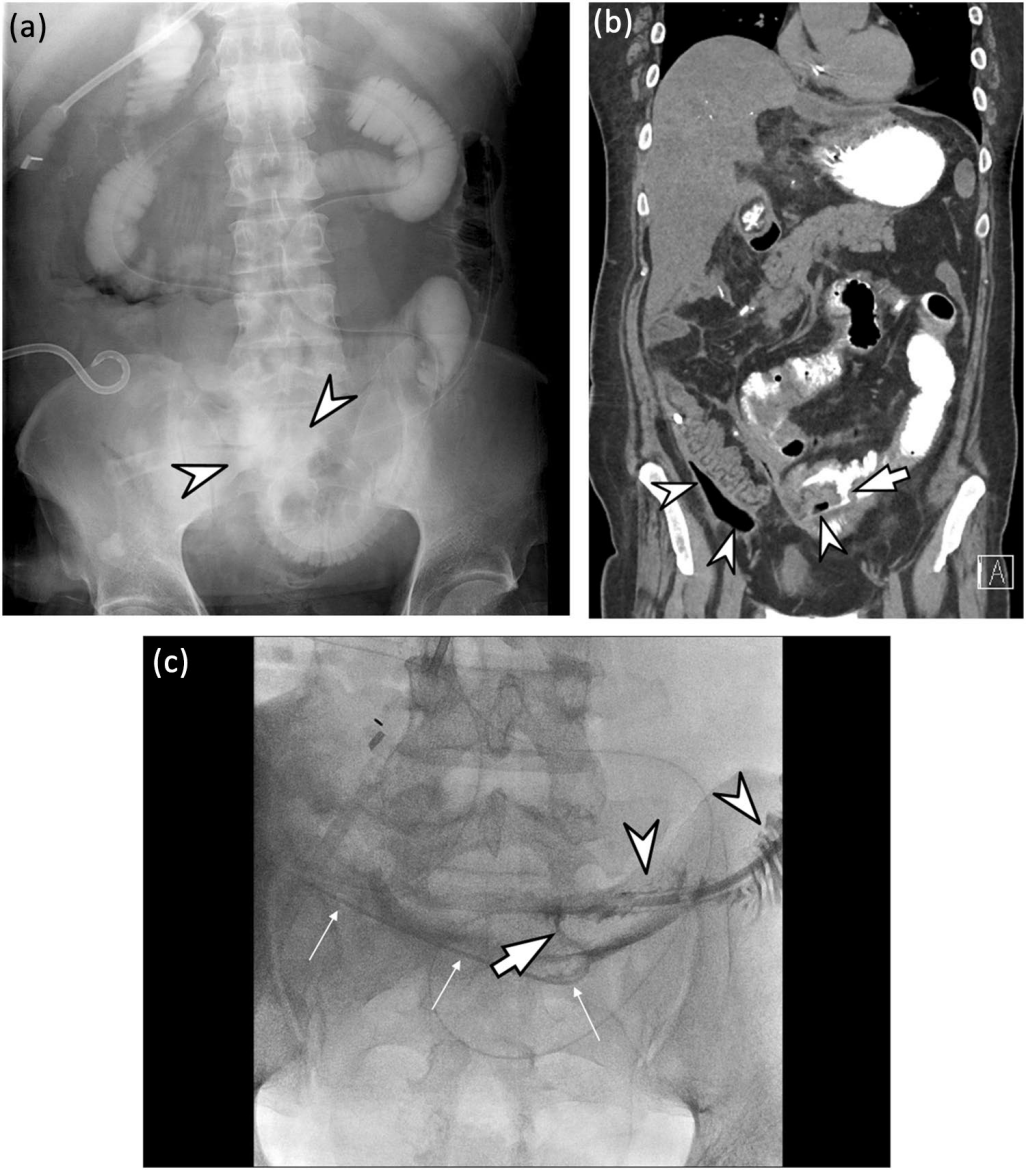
A 40-year old man who underwent primary repair operation for small bowel perforation. After operation, the patient complained of abdominal pain and persistent fever. (a) On small bowel follow-through examination after taking a 100 ml of oral iodinated contrast agent (gastrografin®), a fuzzy-marginated, increased attenuating area (arrowheads) in the pelvic cavity is observed. However, two radiologists were not able to determine the presence of leakage and to localize the leakage site. Therefore, they scored 3 (possibly present) for the presence of leakage. (b) On a coronal non-contrast CT image obtained immediately after fluoroscopic examination, a small & mild defect (arrows) is clearly demonstrated at the inferior aspect of ileum. There is also a moderate amount of air-containing cavity with extravasated contrast material (arrowheads) around the leakage site. Both radiologists scored 5 (definitely present) for the presence of leakage. (c) On a tubogram obtained after contrast injection through a percutaneous catheter drainage (PCD) tube (thin arrows), a small defect of the ileal wall (arrow) is well demonstrated and the ileal lumen is filled with inserted contrast materials (arrowheads).
